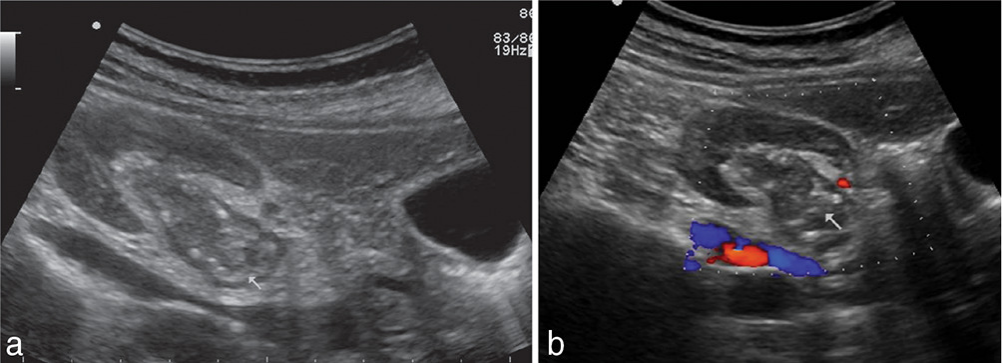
Ultrasonogram (a) and colour Doppler (b) images of the right kidney showing an isoechoic lesion (white arrows) in the renal pelvis with a peripheral rim of calcification and absence of vascularity.
